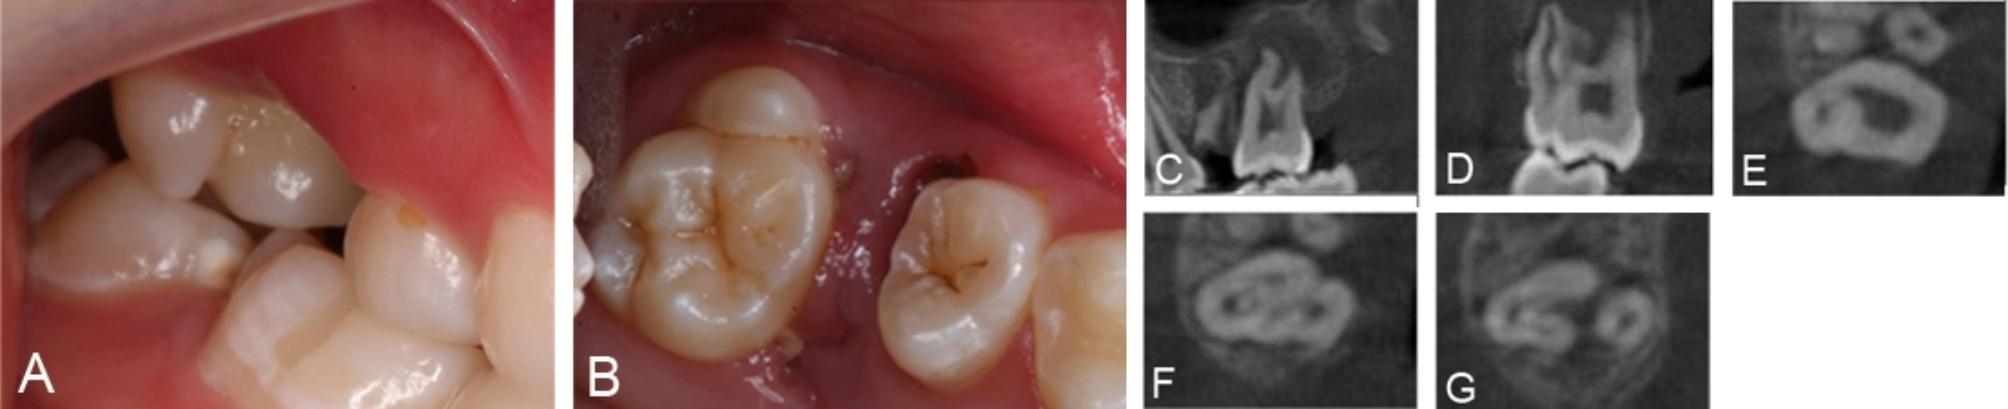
Preoperative intraoral photographs and radiographs of the fusion tooth in case 3. A,B: Buccal and occlusal intraoral view. The occlusal surface shows four intact cusps, in addition to one relatively independent cusp close to the buccal side of the crown, and the crown is free of caries, cracks or dental defects; C-D: CBCT sagittal and coronal cross-sections of the fused tooth, showing that the root canal system of the second molar and the supernumerary tooth were not connected to each other; E-G: CBCT axial cross-sections of the fused tooth.The root canal systems of the affected tooth and the multiple teeth remain independent from the crown to the root apex
